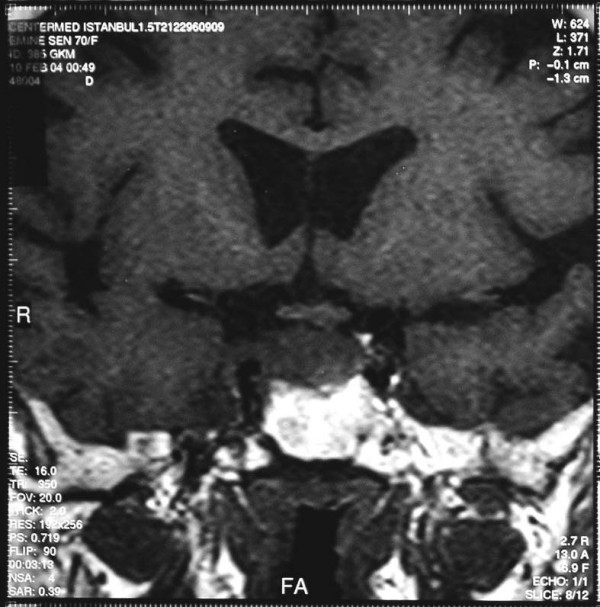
Precontrast coronal pituitary magnetic resonance imaging T1W1.
